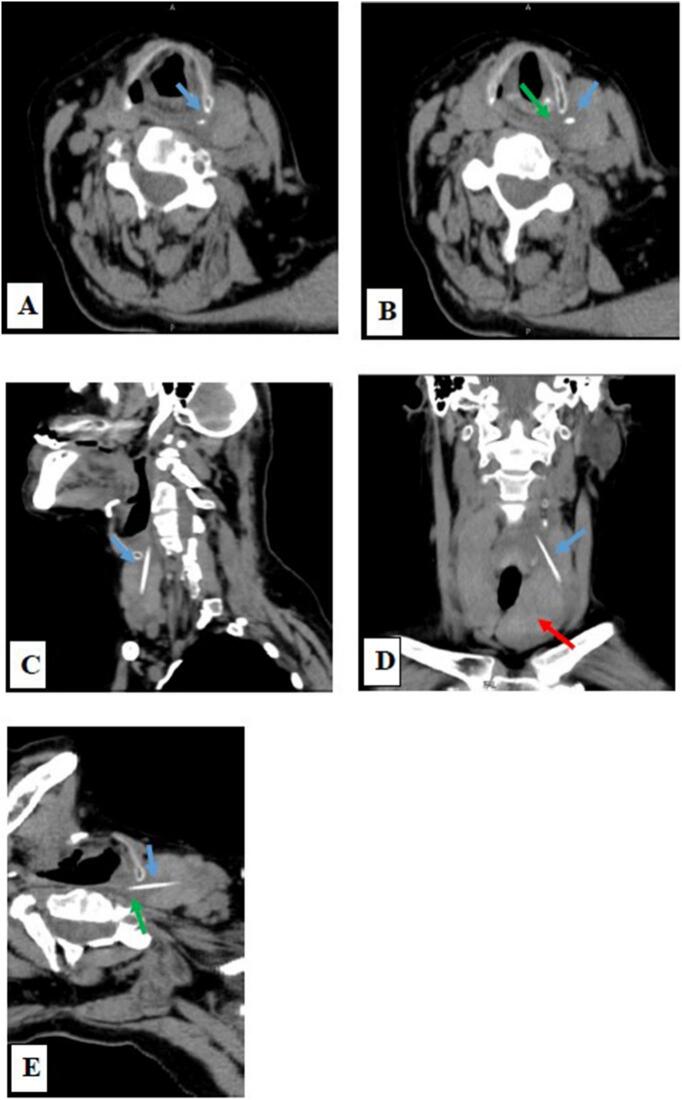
Multiplanar CT images for the neck without IV contrast in the axial section (A and B) revealed a radiodense foreign body shown in Fig. 2. (blue arrow) lodged in the left lobe of the thyroid gland, its upper end abutting the left lateral wall of the hypopharynx at C5 vertebral body level which showed surrounding hypodensity representing the inflammatory reaction of surrounding soft tissue (green arrow) and lower end in the left lobe of the thyroid, no abscess collection or emphysema was noted. C and D: Sagittal and coronal section showed the linear radiodense foreign body (blow arrow) lodged in the left thyroid lobe; the Thyroid gland is enlarged and shows features of multinodular goiter (red arrow). E: The reformatted axial image showed the full length of the foreign body (blue arrow) with surrounding hypodensity along its course representing the inflammatory reaction of surrounding soft tissue (green arrow). (For interpretation of the references to colour in this figure legend, the reader is referred to the web version of this article.)
